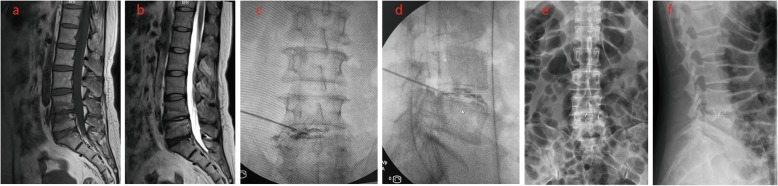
Modic changes type I was characterized by low T1 and high T2 signals in MRI on L4-5 endplate (a, b). The patient experienced severe LBP and confirmed to be discogenic by discography (c, d). After the discoblock was administered, the patient complained LBP relieved. The patient had reoccurring pain and underwent OLIF after 3 months follow-up (e, f)
